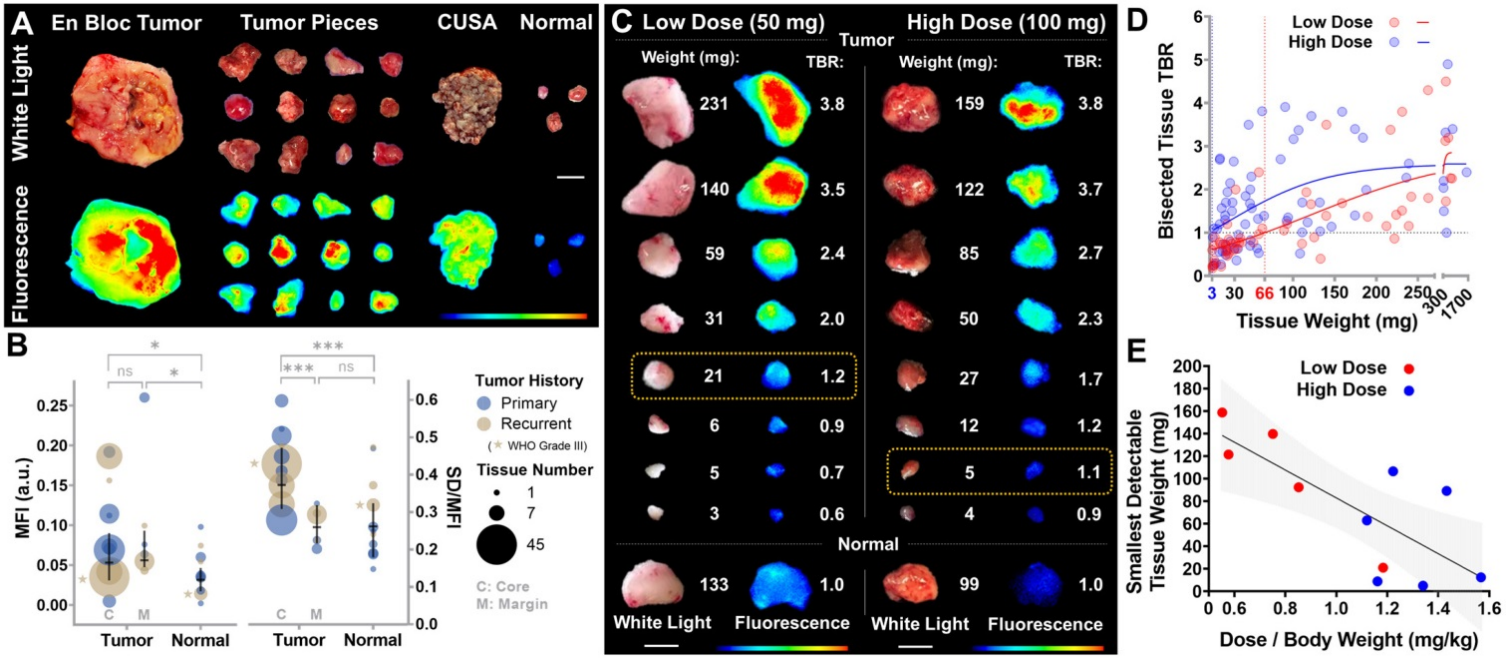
Detection thresholds of intraoperative NIR fluorescence imaging *ex vivo*. (A) Intraoperative white light photographs and corresponding closed-field NIR fluorescence images of resected tissue. CUSA: cavitron ultrasonic surgical aspirator. Scale bar = 1 cm. (B) Average mean fluorescence intensity (MFI) and fluorescence heterogeneity (standard deviation normalized to mean fluorescence intensity) in tumor and normal tissue pieces. Each circle represents one patient. MFI: *P* = 0.7407 (C vs M), * *P* = 0.0364 (C vs Normal) and * *P* = 0.045 (M vs Normal); SD/MFI: * *P* = 0.0003 (C vs M) and * *P* = 0.0001 (C vs Normal) by ANOVA with multiple comparisons. (C) Postoperative white light photographs and corresponding closed-field NIR fluorescence images of serial bisected fresh tumor tissue *ex vivo*. *Yellow boxes*: smallest detectable tumor tissue in each dose cohort. Scale bars = 1 cm. (D) Target-to-background ratios (TBR) of resected tissue fluorescence is plotted against tissue weight of each dose cohort (each symbol represents one tumor piece). *3 mg and 66 mg:* intersections of fitted curves with TBR = 1. (E) The smallest detectable tumor tissue weight correlated with body weight adjusted panitumumab-IRDye800 dose. *P* = 0.007 (each symbol represents one patient, simple linear regression goodness of fit: R^2^ = 0.57).
